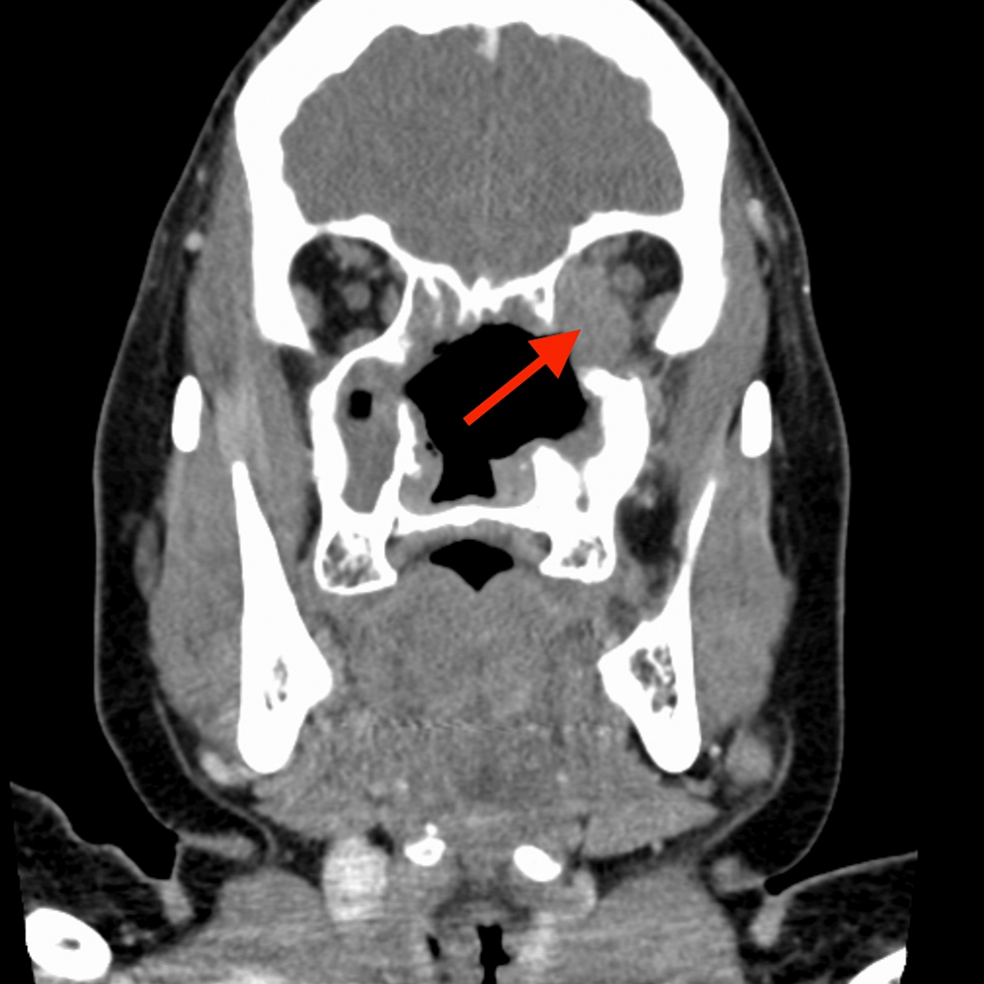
CT facial bones with contrast coronal CT: computed tomography
